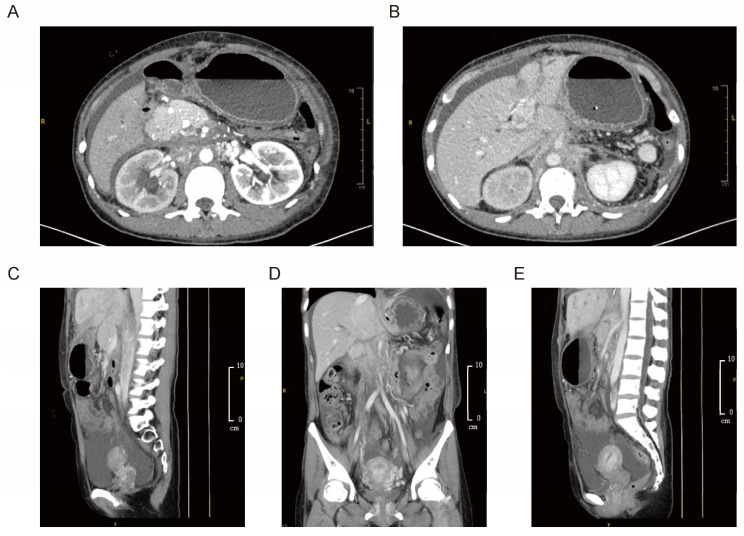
Imaging findings of retroperitoneal fibrosis with peripancreatic and hepatic involvement of the patient. (A,B) Abdominal contrast-enhanced CT revealed duodenal compression by a congenitally enlarged pancreatic head, stricture of the hepatic portal bile duct, and dilation of second- and third-order bile ducts on the right. The bile duct wall appeared thickened with contrast enhancement. (C–E) Coronal and sagittal contrast-enhanced CT demonstrated thickening and enhancement of the bile duct wall. Space-occupying lesions in the retroperitoneal and peripancreatic regions extended toward the round ligament of the liver, exhibiting a fibrosis-like appearance rather than a well-defined mass.
